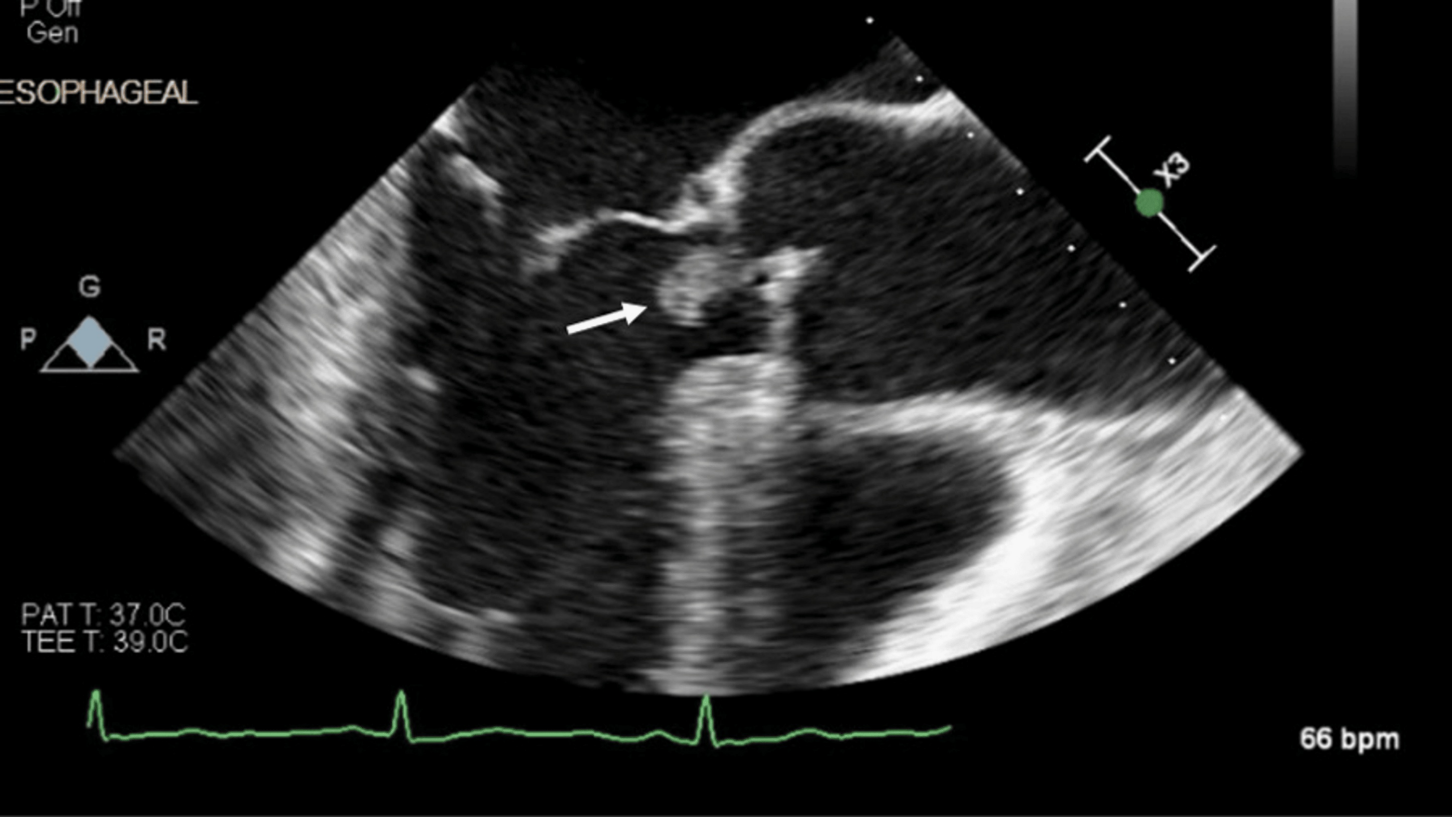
TEE showing a >10-mm mobile density on the noncoronary cusp in the mid-esophageal long-axis view TEE, transesophageal echocardiogram
